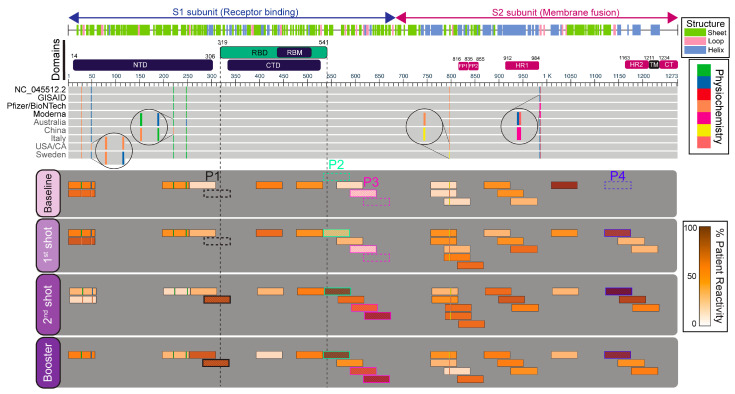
Epitope map of anti-S responses represented as percent subject reactivities. The multiple sequence alignment (MSA) of full-length SARS-CoV-2 spike glycoprotein reference, vaccine, and input library sequences are shown, where regions in light grey represent sequence conservation. Mismatched residues are highlighted and color-coded using the pre-defined Zappo scheme (green: hydrophilic, salmon: aliphatic/hydrophobic, orange: aromatic, fuchsia: conformationally special, yellow: cysteine only, red: negatively charged, blue: positively charged). Reactive peptides are mapped and colored based on % subject reactivity (brown gradient) across timepoints. Vaccine-boosted epitopes are indicated (P1–P4). Peptides with transparent backgrounds and dashed borders indicate absence of baseline responses and are only shown for convenience. Abbreviations: NTD: N-terminal domain, CTD: C-terminal domain, RBD: Receptor-binding domain, RBM: Receptor-binding motif, FP1/2: Fusion peptide 1/2, HR1/2: Heptad repeat 1/2, TM: Transmembrane, CT: Cytoplasmic tail.
